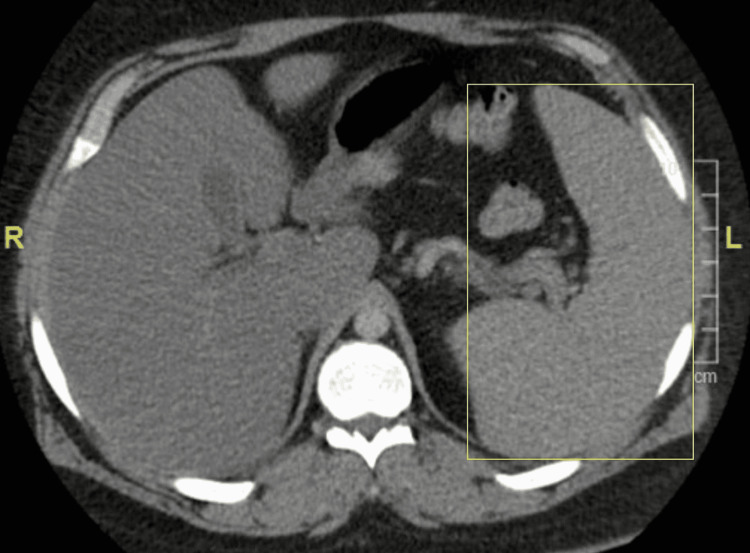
Axial view of abdominal CT angiography showing splenomegaly Enlarged spleen measuring 18 cm shown within the yellow borders.
